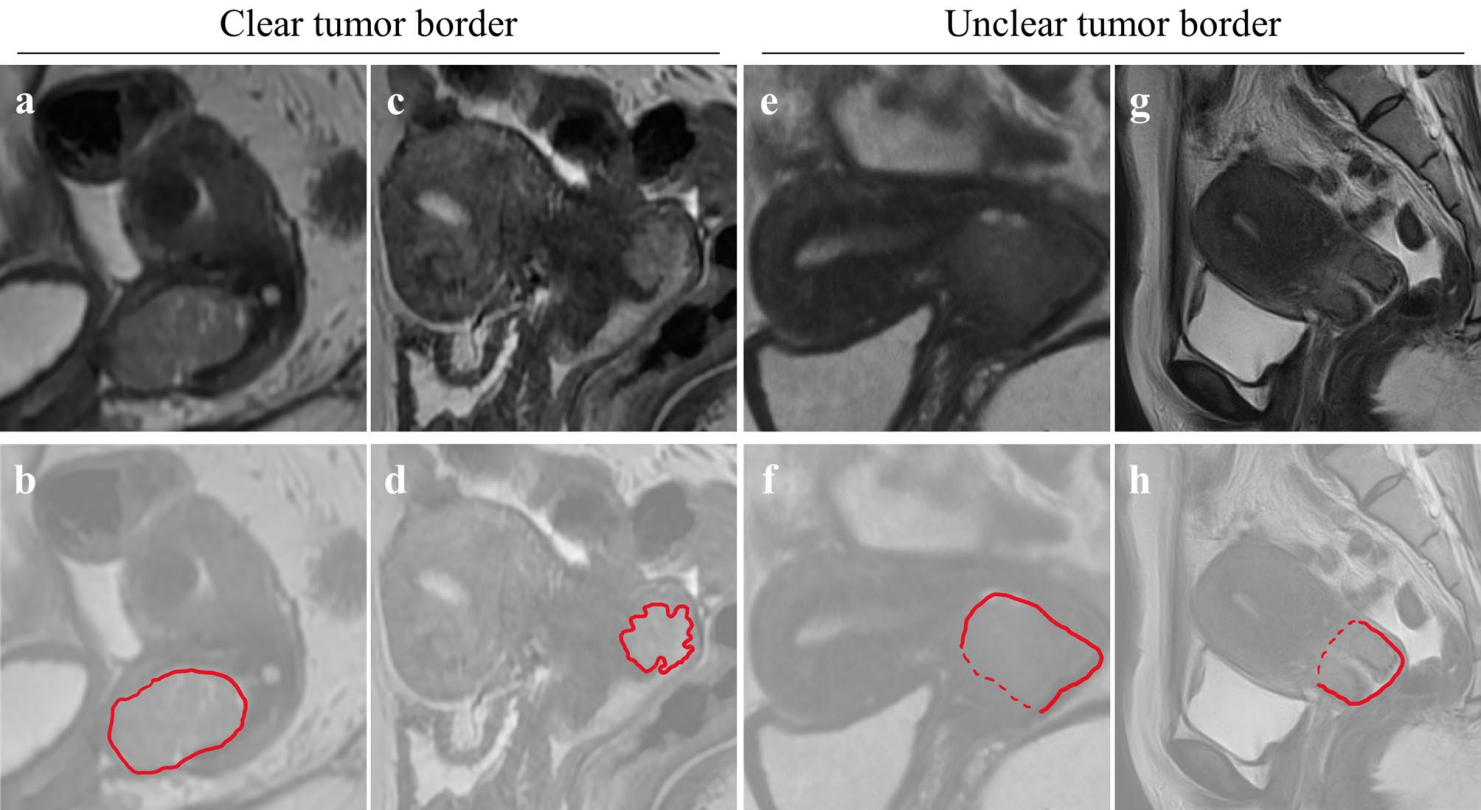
Example of classification based on the tumor border using magnetic resonance imaging. (a,b) A 60-year-old patient with a clear tumor border. The solid line indicates the clear borderline of the tumor. (c,d) A 46-year-old patient with a clear tumor border. The solid line indicates the clear borderline of the tumor. (e,f) A 29-year-old patient with an unclear tumor border. The solid line indicates the clear borderline, while the dotted line indicates the unclear borderline of the tumor. (g,h) A 35-year-old patient with an unclear tumor border. The solid line indicates the clear borderline, whereas dotted line indicates the unclear borderline of the tumor.
